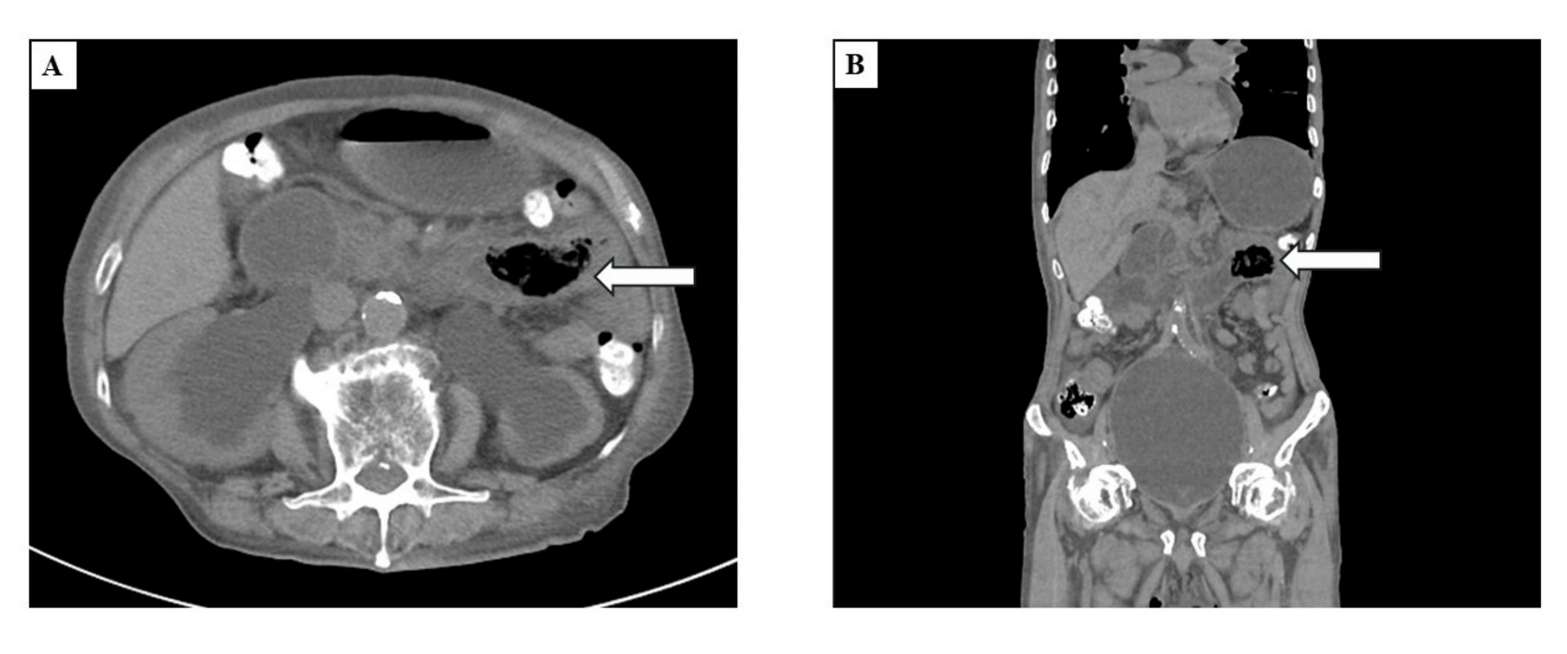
Computed tomography (A: axial view; B: coronal view) showing bilateral hydronephrosis and a massive gas-containing structure in the lumen of the proximal jejunum (arrow)
